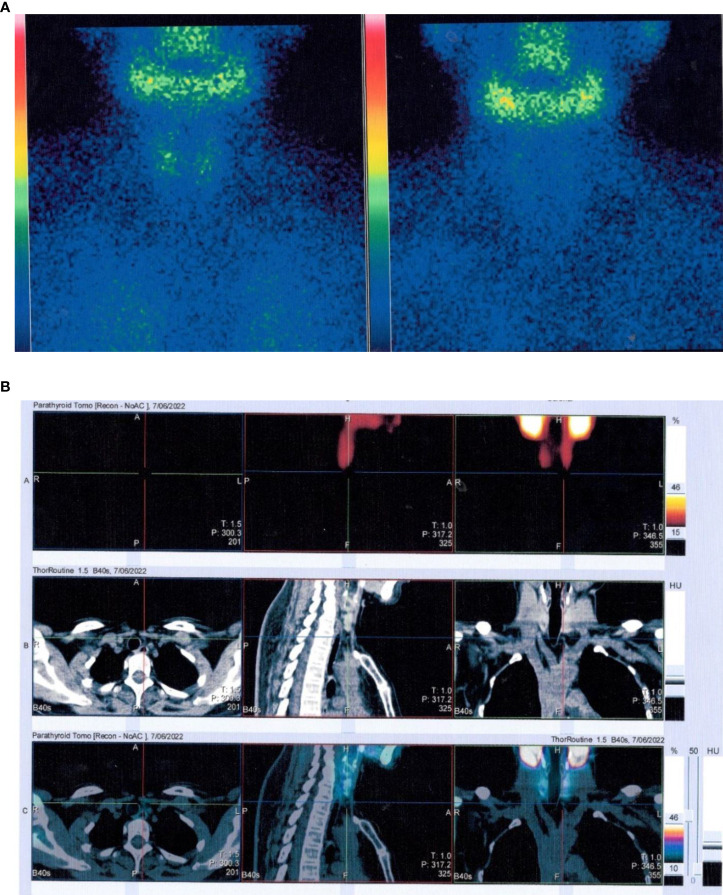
Emission computed tomography of the parathyroid gland. (A) No radioactive uptake in the thyroid/parathyroid gland. (B) The nodule (8 mm * 3 mm * 5 mm) in the lower pole of the left lobe of the thyroid gland did not uptake radiation.
